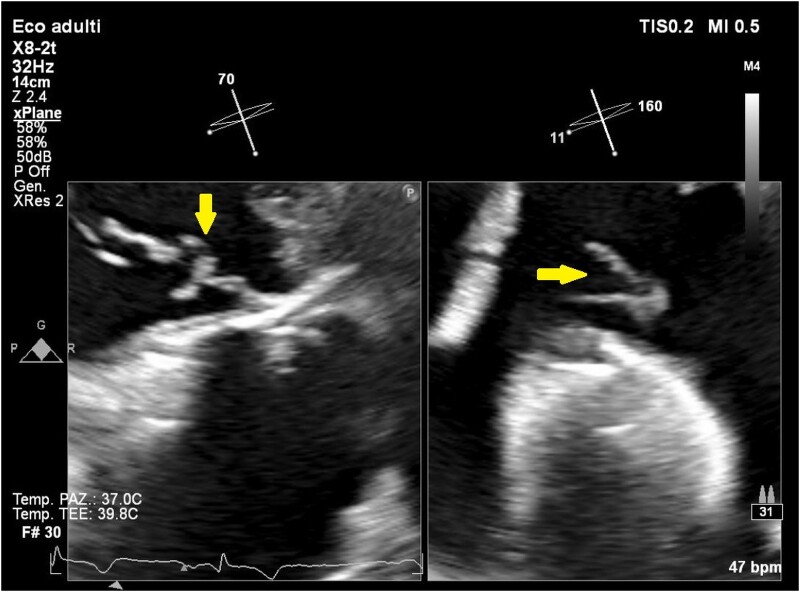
Zoomed off-axis short axis view of the tricuspid valve from the transoesophageal transgastric window with X-plane imaging, showing a ‘finger-in-glove’ and ‘garland-like’ appearance with adherent filaments resembling second-order chordae tendineae.
